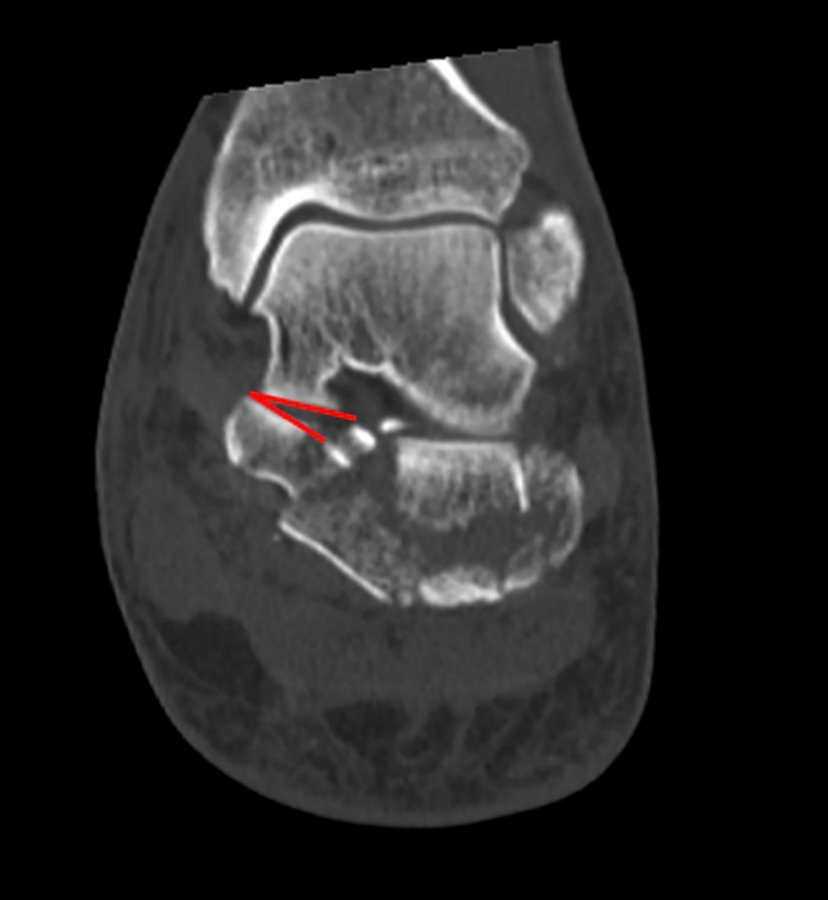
Semicoronal CT section. Fracture of the calcaneus involvement of the sustentacular fragment. Note that the sustentacular fragment is both tilted and fractured. The angle between the talar and calcaneal facets of the middle subtalar joint is 27°
